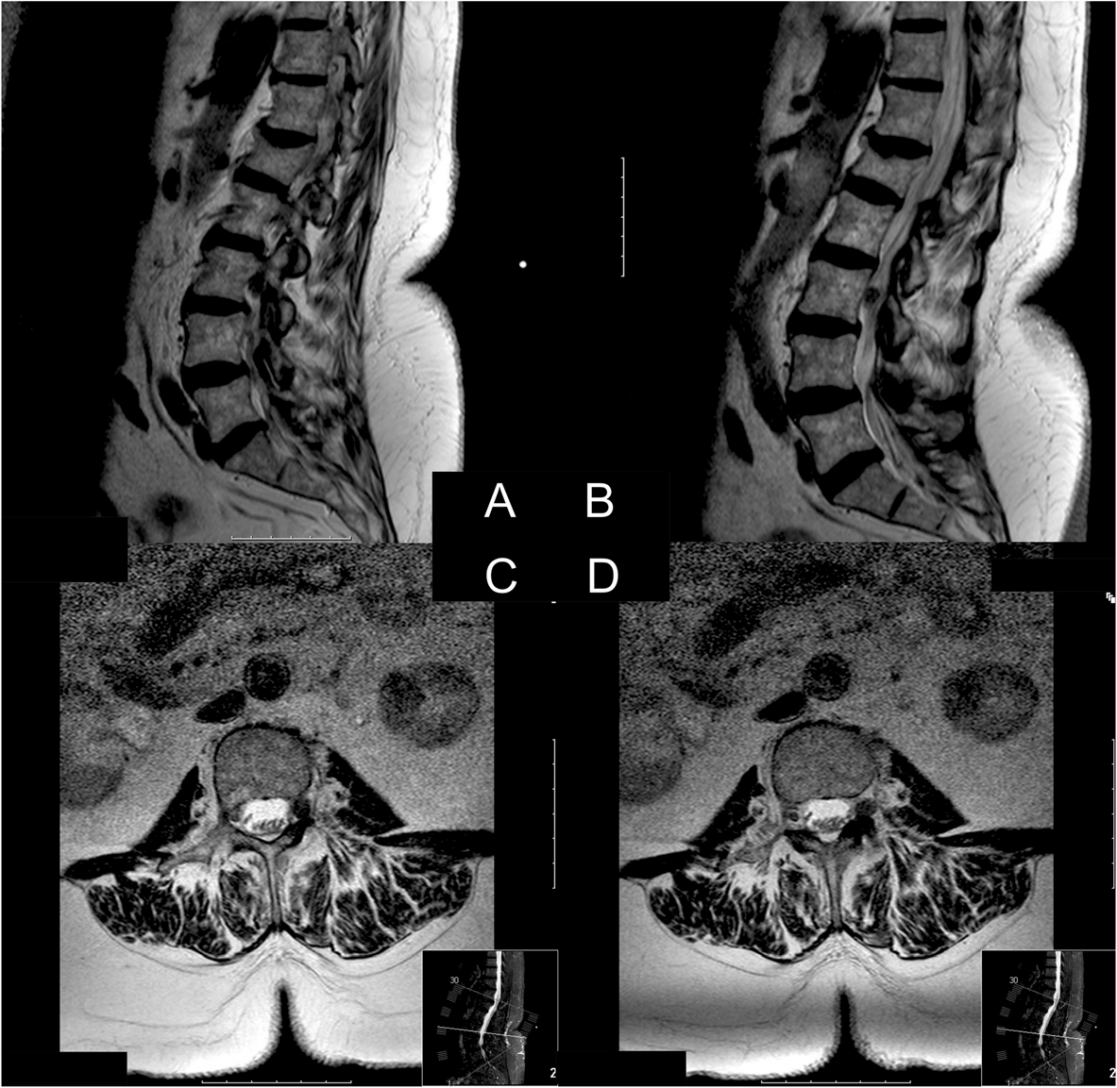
Preoperative magnetic resonance imaging. (A) Sagittal non-enhanced T1-imaging. (B) Sagittal T2-imaging. (C, D) Axial T2-imaging. An unclear structure in the neuroforamen of the L3 left nerve root not clearly delimitable. There was no clear connection to the intervertebral disc space. No gadolinium was applied because of renal insufficiency.
